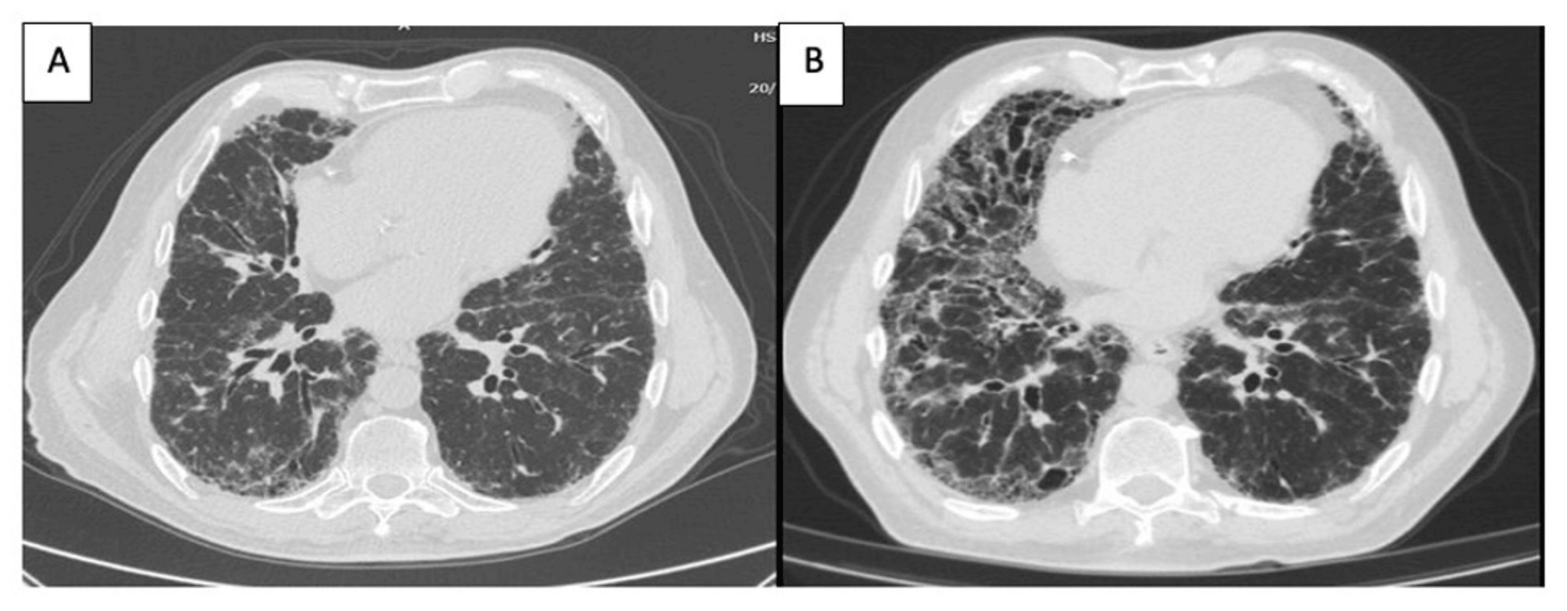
HRCT of a case with HP: (A) image during the initial evaluation and (B) after two years of follow-up. The images show a significant progression of the fibrotic pattern, increased ground glass, and increased traction bronchiectasis HRCT: high-resolution computed tomography; HP: hypersensitivity pneumonitis
